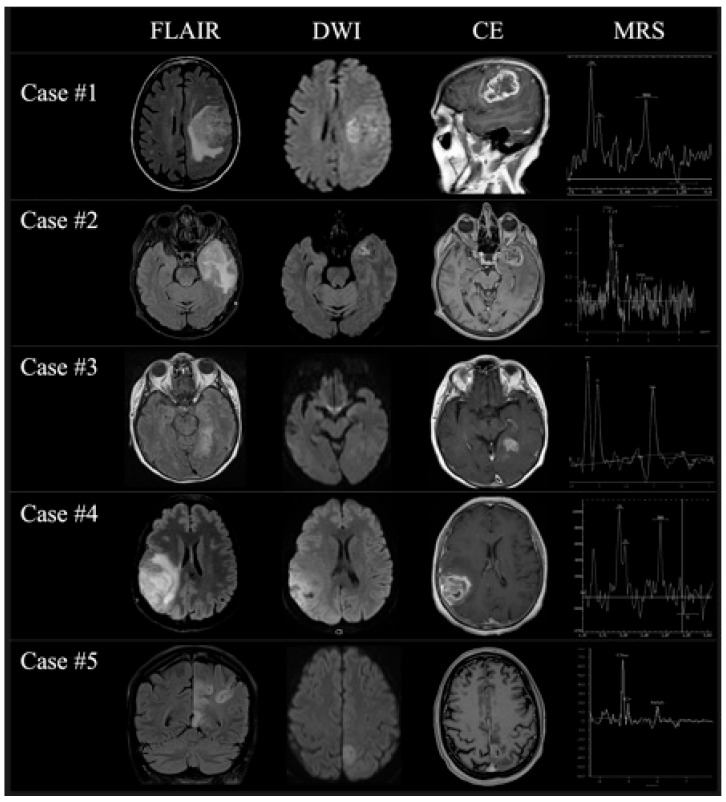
A panel showing the most relevant MRI changes of all patients at the second time-point MRI examination on FLAIR (first column), DWI (second column), contrast-enhanced T1w (third column), and MRS (fourth column) once the diagnosis of GBM was finally considered.
